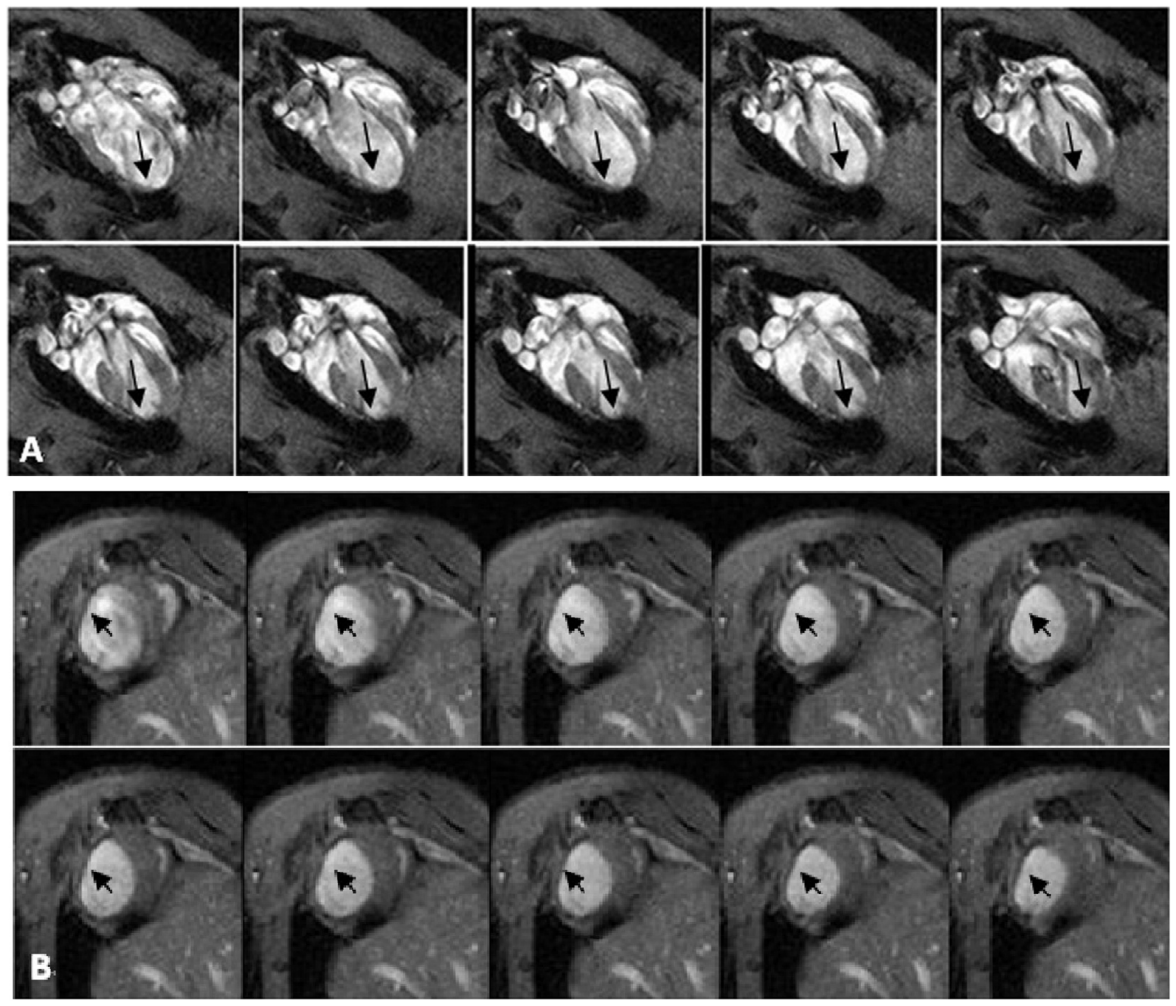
Cine MR imaging performed 2 weeks after MI. The four-chamber views (A) and short-axis views (B) showed that the anterior wall of left ventricle became thinner, the motion of the anterior and apical wall decreased or disappeared, and aneurysm appeared after myocardial infarction.
